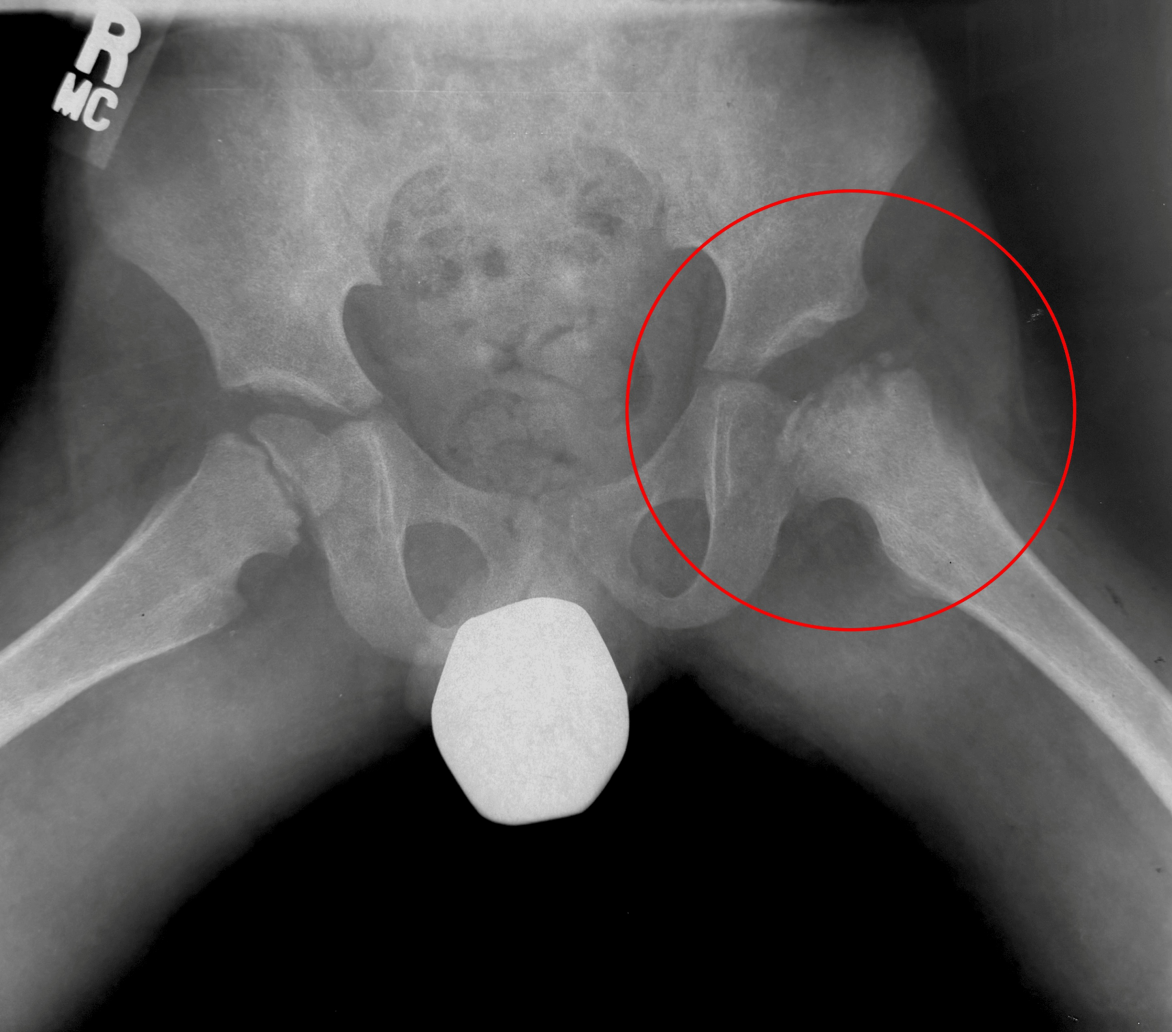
Frog leg lateral view pelvic X-ray taken on March 1, 2007.
